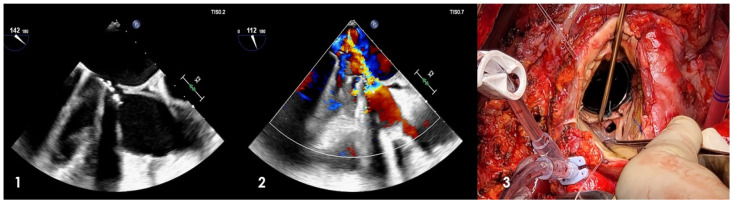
Aortomitral curtain disjunction in double mechanical prosthesis infective endocarditis. (1,2) Transesophageal echocardiogram imaging showing communication and jet from the ascending aorta to the left atrium; (3) intraoperative founding of aortomitral curtain disjunction detected through the aortotomy (*black hole*) (Cardiovascular Department, UO of Cardiac Surgery of IRCCS Humanitas Research Hospital).
